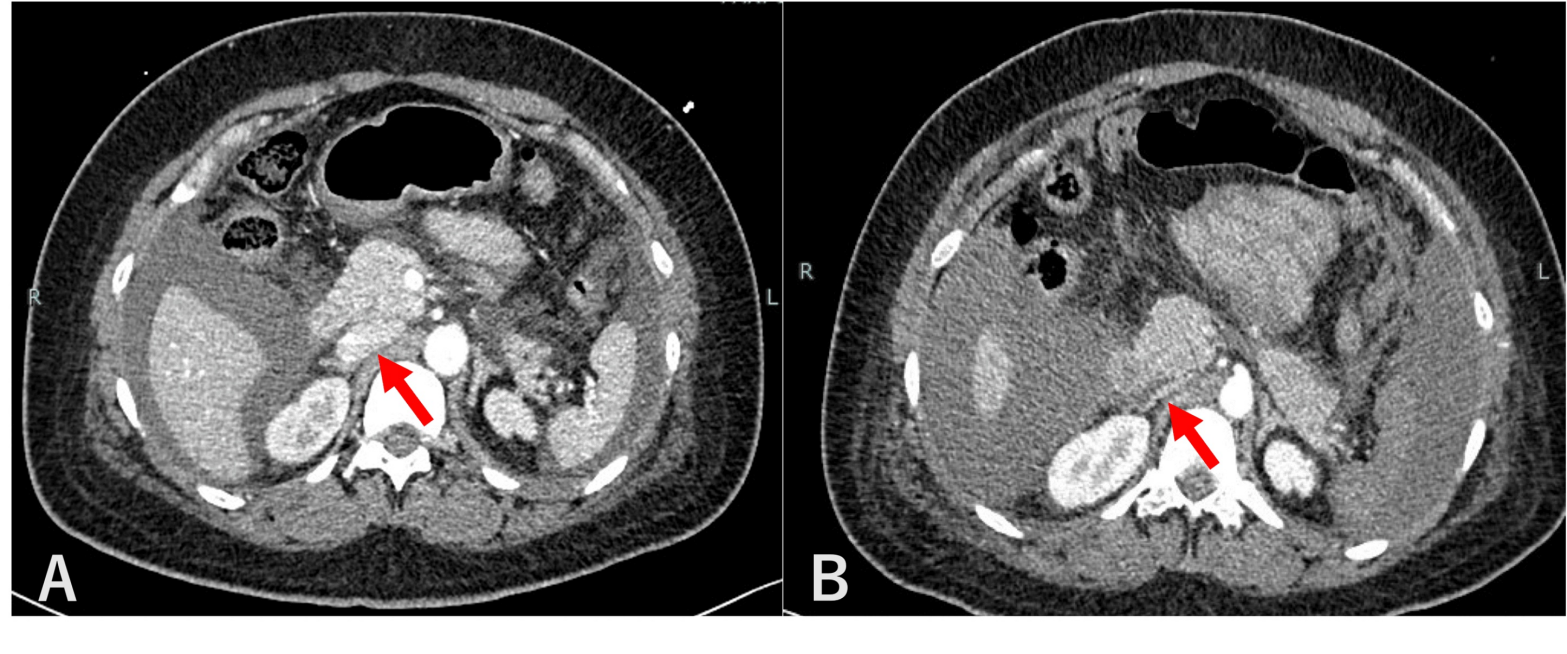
Comparison CT images of the inferior vena cava. (A) Image of the day before. (B) Image at cardiopulmonary arrest.
